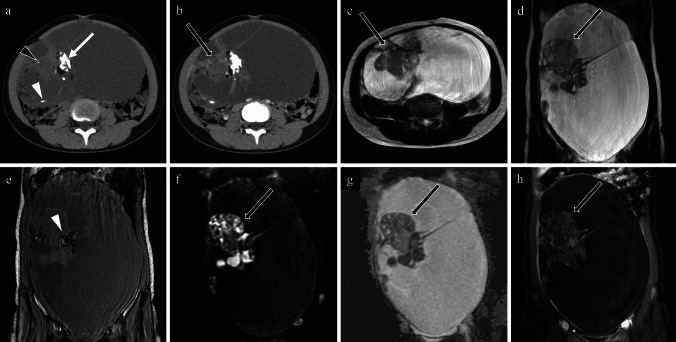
18-year-old woman with an immature teratoma of the left ovary. a non-contrast CT; b contrast-enhanced CT; c axial T2-weighted image; d coronal T2-weighted image; e coronal T1-weighted image (opposed-phase chemical shift image); f coronal diffusion-weighted image; g
